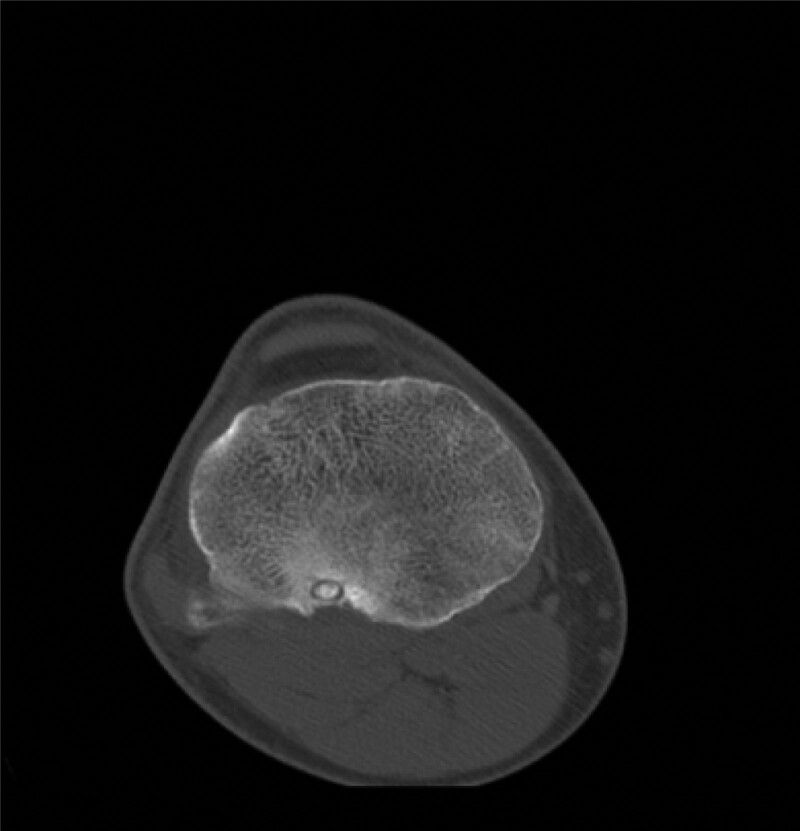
Preoperative CT image in transverse plane. CT = computed tomography.
